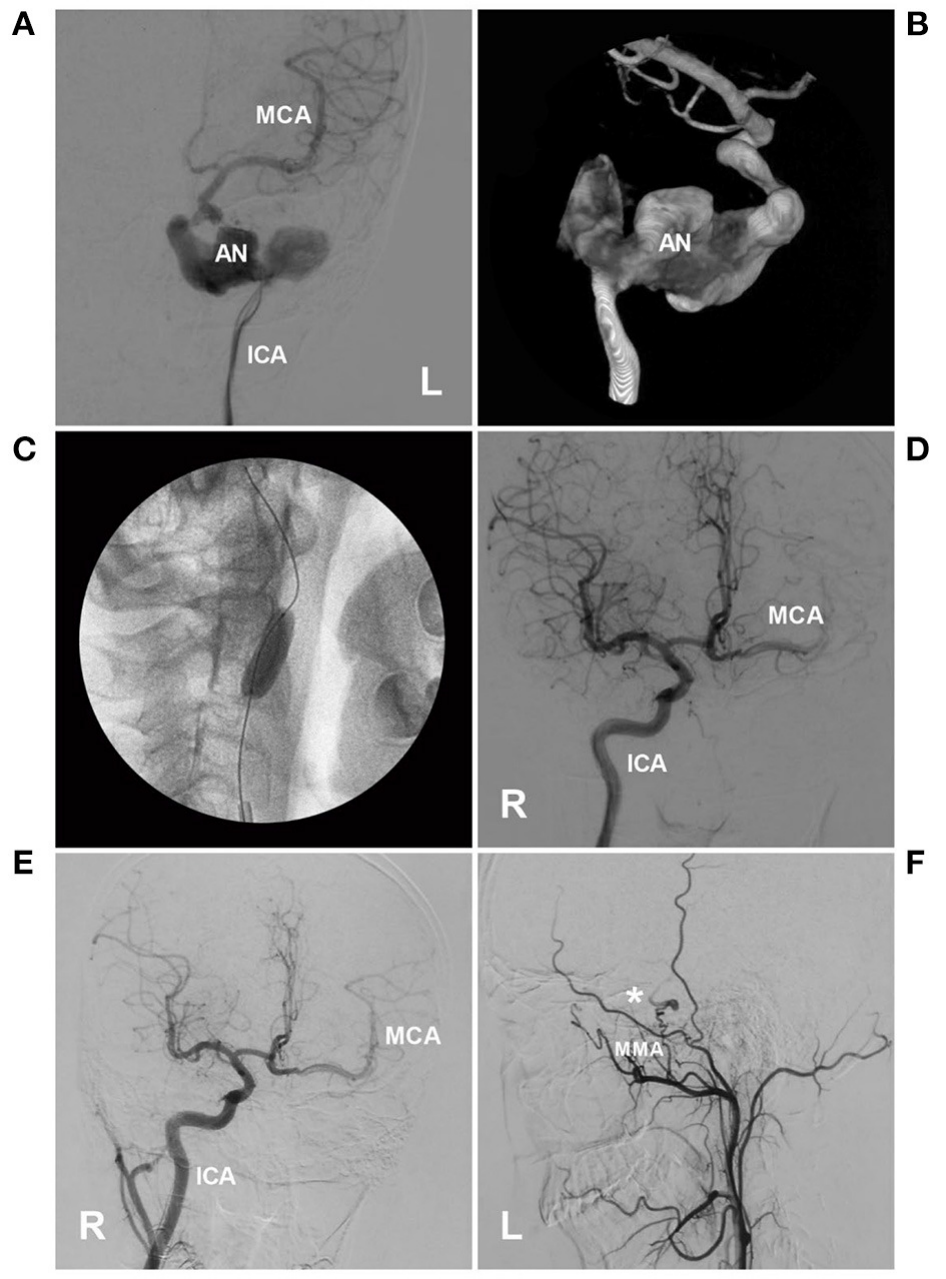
PAO of the ICA for a giant petrous segment aneurysm. (A,B) Left DSA (A) and three-dimensional DSA (B) showed an unregular giant aneurysm (AN) on the petrous segment of the ICA. (C) X-ray film showed that the BOT was performed, and the balloon was located in the ICA under the aneurysm. (D) During the BOT, DSA of the right ICA showed collateral circulation through the AcomA to the left MCA. (E) After PAO of the left ICA, 1-year follow-up DSA showed that collateral circulation through the AcomA was sufficient. (F) The external carotid artery showed anastomosis between the MMA (asterisk) and intracranial pial artery. AcomA, anterior communicating artery; BOT, balloon occlusion test; DSA, digital subtraction angiography; ICA, internal carotid artery; L, left; MCA, middle cerebral artery; MMA, middle meningeal artery; PAO, parent artery occlusion; VA, vertebral artery.
